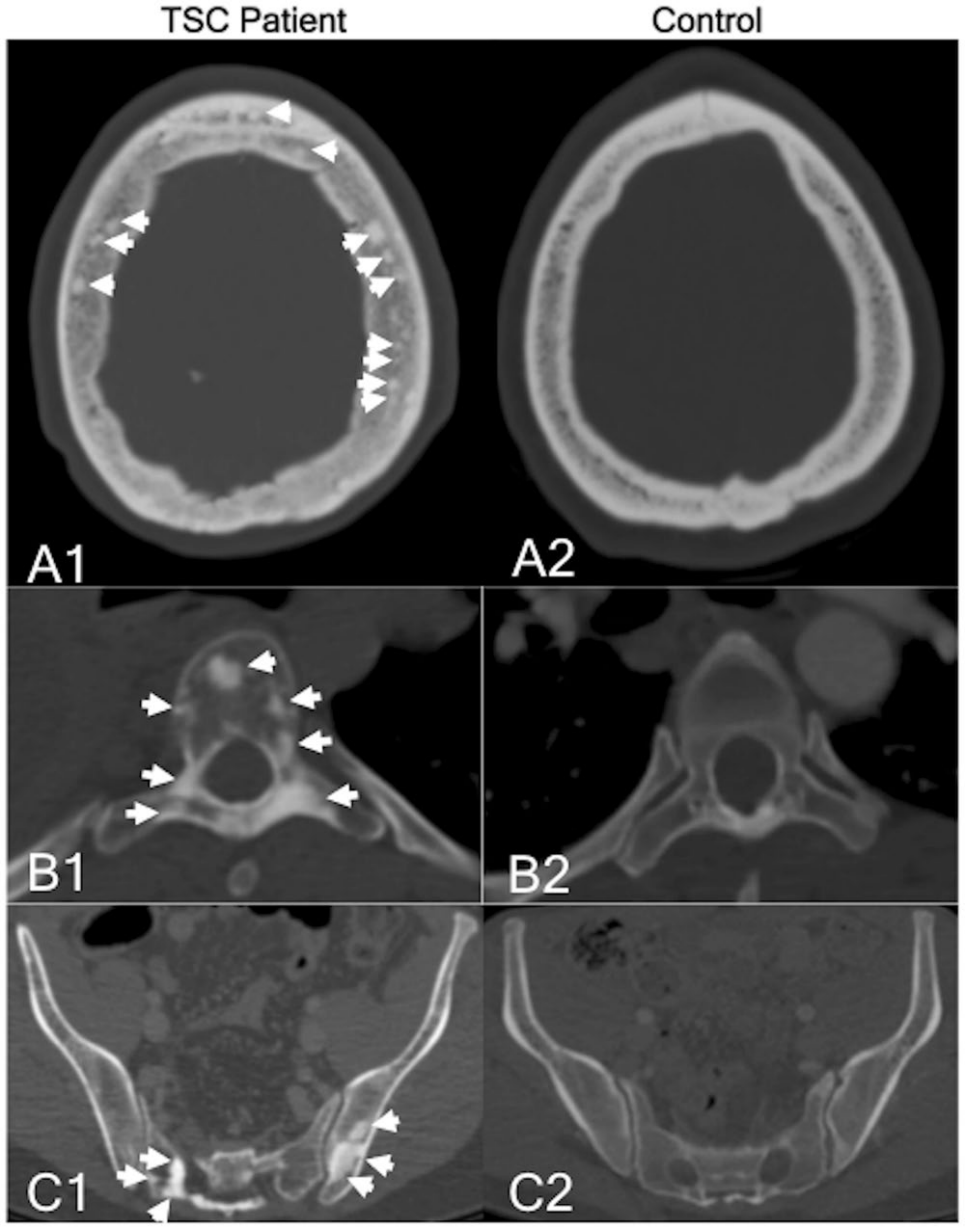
CT imaging example of the location pattern of sclerotic bone lesions in the skull, spine, and pelvis of TSC patients and control subjects. (A1,A2) Transversal CT of the skull of a TSC patient and a control subject. (A1) A relatively high number of SBLs (indicated by white arrows) can be seen in the spongiosa of the skull. The highest number of SBLs can be found in the os parietale and the os frontale. (A2) Normal spongiosa of a control individual without focal sclerotic bone lesions. (B1,B2) Transversal CT of the spine in a TSC patient and a control subject. (B1) The highest number of SBLs (indicated by white arrows) can be detected in the thoracic spine in the vertebral body and the arcus vertebrae/processus spinosus. Other osseous structures (e.g. sternum or ribs) were also affected, but to a lesser degree. (B2) Normal spongiosa of a control individual without focal sclerotic bone lesions. (C1,C2) Transversal CT of the pelvis in a TSC patient and a control subject. (C1) In the abdomen/pelvis, the second-highest number of SBLs (indicated by white arrows) could be detected in the pelvis. The highest number could be detected in the spine (example please see Fig. 2, B1). Other osseous structures such as the femur were also affected, but to a lesser degree. (C2) Normal spongiosa of a control individual without focal sclerotic bone lesions.
